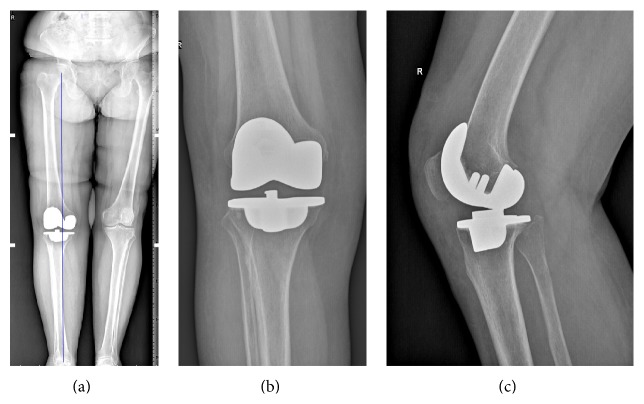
Long standing anteroposterior X-ray showing adequate anatomic alignment (a) and anteroposterior and lateral nonweight bearing radiographs ((b) and (c), resp.).
